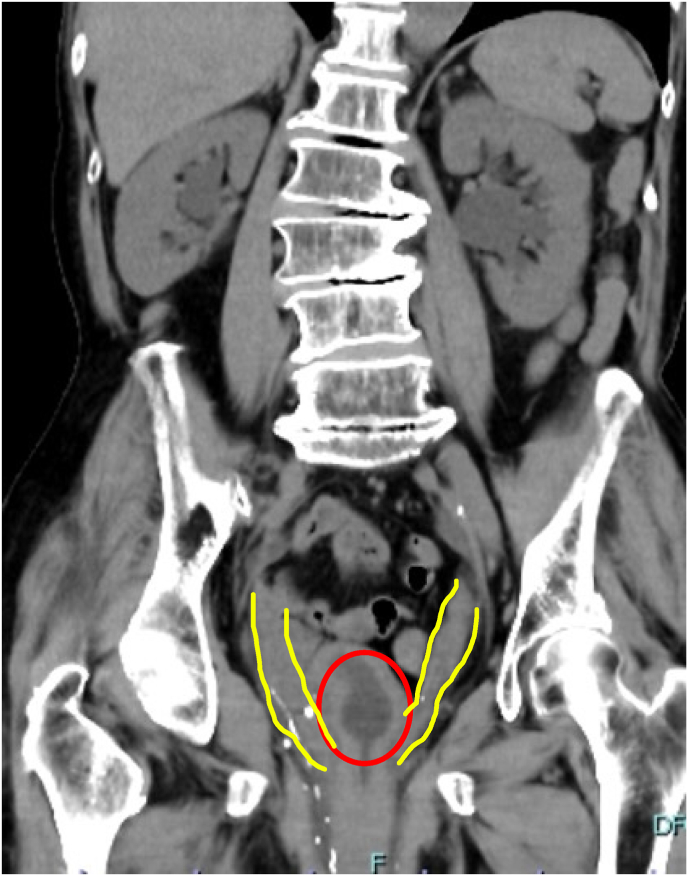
Coronal section of CT. Both ureters are dilated (yellow lines). The balloon in the bladder is apparent on computed tomography (red circle). (For interpretation of the references to colour in this figure legend, the reader is referred to the Web version of this article.)
